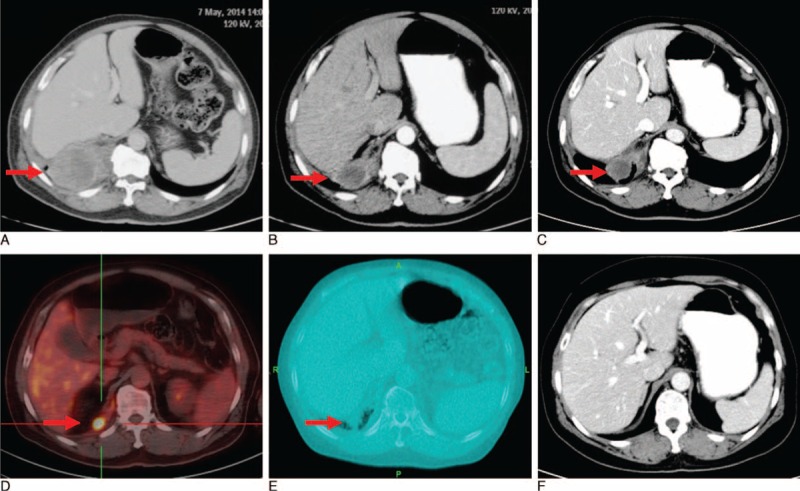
(A) Residual disease was also demonstrated by CT before the initial chemotherapy. (B) After 2 cycles of fist-line chemotherapy, the disease was partial response. (C) After 4 cycles of fist-line chemotherapy, the disease was partial response. (D) After 6 cycles of fist-line chemotherapy, the disease was stable. (E) The disease remained stable after radiotherapy. (Note: this picture is captured by megavoltage CT (MVCT) in tomotherapy system at the time of the last irradiation.) (F) After 6 course of pemetrexed maintenance therapy (PMT), the residual mass disappeared. CT = computed tomography.
